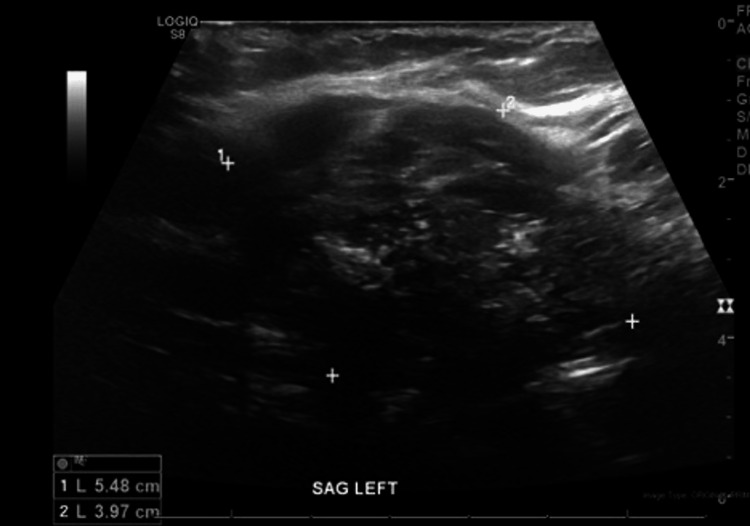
Thyroid ultrasound (sagittal view) of the left thyroid lobe with a solid, hypoechoic, irregular, heterogenous nodule with punctate echogenic foci, measuring 5.5 x 4.0 cm with extrathyroidal extension, suspicious for malignancy.
